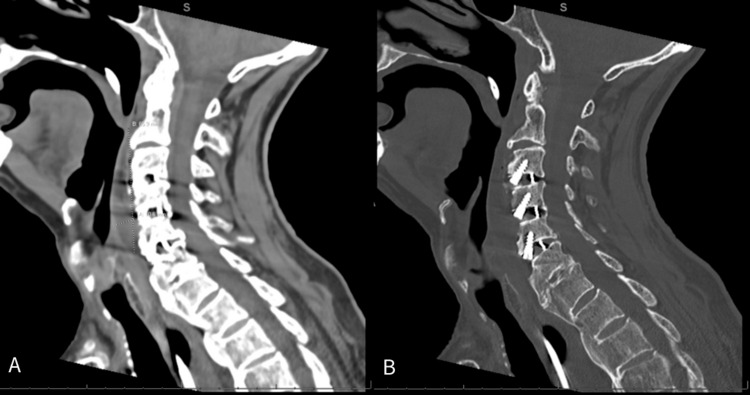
(A and B) Postoperative sagittal CT of the cervical spine demonstrating restoration of normal cervical vertebral alignment and anatomy. Original CT image from Royal Hobart Hospital, Australia, 2025, reproduced with permission from Royal Hobart Hospital Radiology Department
